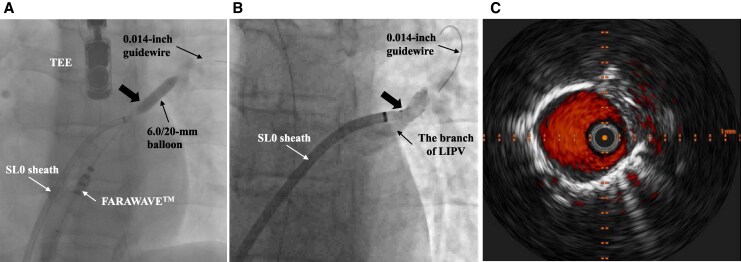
(*A*) Balloon-assisted guidewire extraction at the site of entrapment (arrow) in the left inferior pulmonary vein. A 6.0/20-mm balloon was inflated adjacent to the entrapped segment of the guidewire to stabilize the vessel wall and mitigate potential vascular injury, while the guidewire was carefully withdrawn under continuous transoesophageal echocardiographic monitoring in a hybrid operating room under general anaesthesia. (*B*) Venography confirmed successful guidewire extraction without evidence of vascular injury (arrow). (*C*) Intravascular ultrasound demonstrated an intact pulmonary venous lumen at the site of prior entrapment. LIPV, left inferior pulmonary vein.
